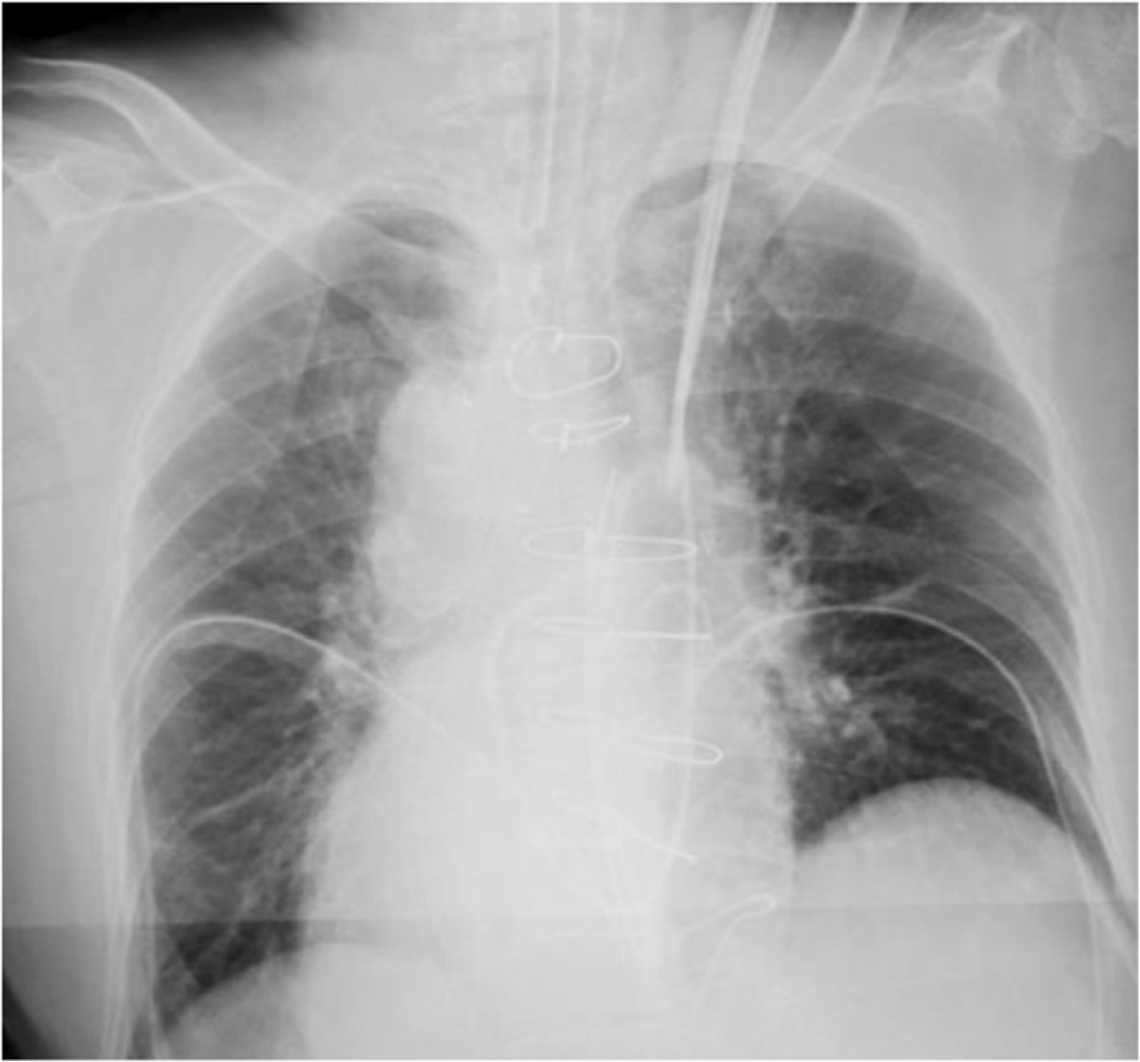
A postoperative chest X-ray revealed that the pulmonary artery catheter was maintained as the mirror image of its normal position
